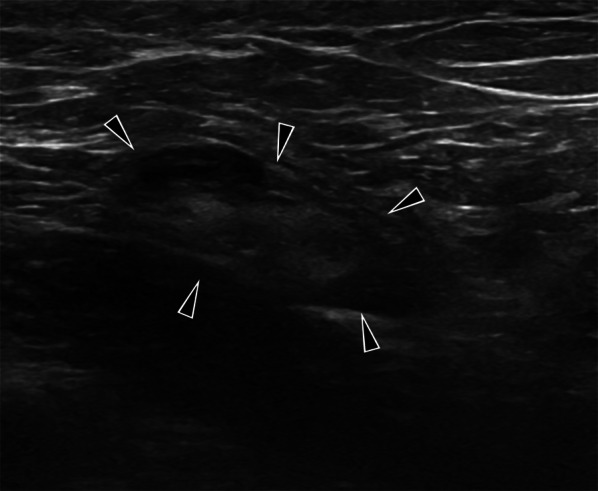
Ultrasound image of a 34-year-old female patient (she had a recently developed clinically suspicious palpable lesion) diagnosed with breast tuberculosis demonstrate nodular tuberculosis (arrowheads). The mass is observed as a well-circumscribed, oval-shaped, heterogeneous solid lesion. BIRADS 4
